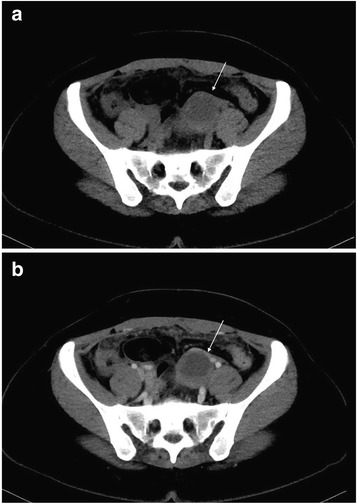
a and b. CT radiographs of the same patient with plain and enhanced imaging. Single arrow in Fig. 6a indicates left ruptured ovarian corpus luteal cyst; single arrow in Fig. 6b indicates the clearer image of the left ruptured ovarian corpus luteal cyst on enhanced CT scan
